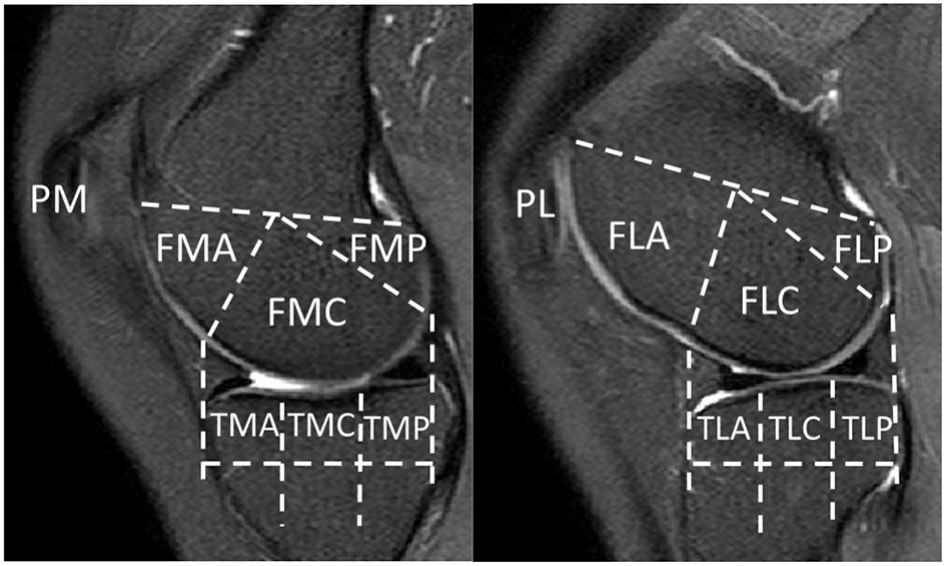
Knee MR images were divided into 14 structural regions-of-interest (ROIs). FMA, medial anterior femur; FMC, medial central femur; FMP, medial posterior femur; FLA, lateral anterior femur; FLC, lateral central femur; FLP, lateral posterior femur; TMA, medial anterior tibia; TMC, medial central tibia; TMP, medial posterior tibia; TLA, lateral anterior tibia; TLC, lateral central tibia; TLP, lateral posterior tibia; PM, medial patella; PL, lateral patella.
